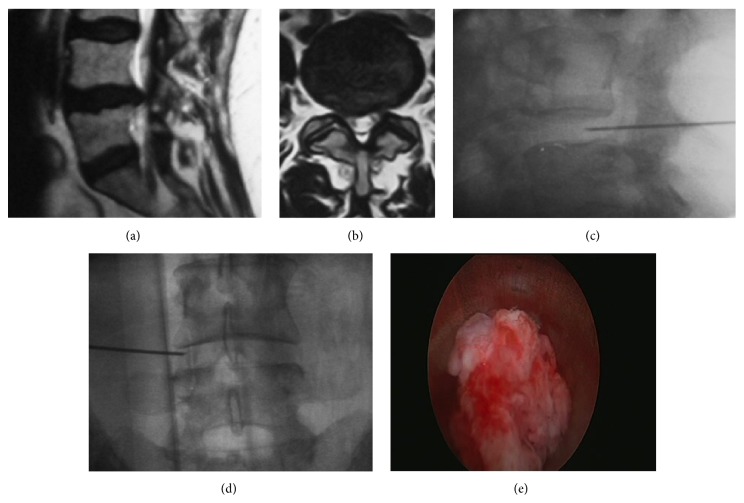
(a-b) Sagittal and axial T2-weighted MRI images of an L4-L5 right disc bulging causing foraminal stenosis. Approach is performed through an entry point located ~7 cm from the midline. (c-d) Intraoperative fluoroscopy and lateral and anteroposterior (AP) projections showing the position of the instrumentation. (e) Fragment of the disc removed from the endoscopic cannula.
